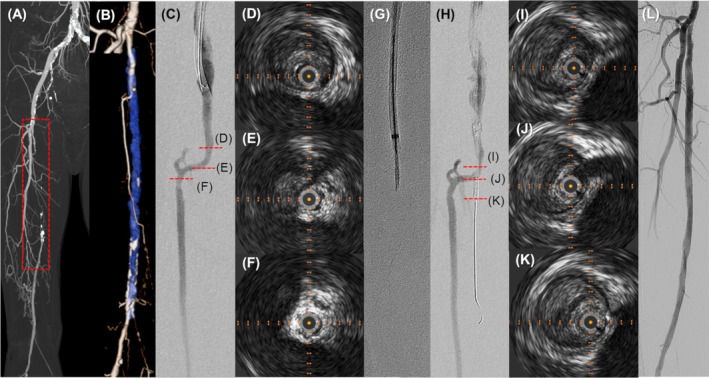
Images of right femoropopliteal artery. (A) CT angiography. (B) Magnified CT angiography with a virtual occlusive vessel image of the area in the rectangle in panel (A). (C–F) Angiography and IVUS findings before using a Wingman catheter. (G) Using a Wingman catheter with a GOGO catheter D‐shape. (H–K) Angiography and IVUS findings after using a Wingman catheter. (L) Angiography after implanting two drug‐eluting stents.
